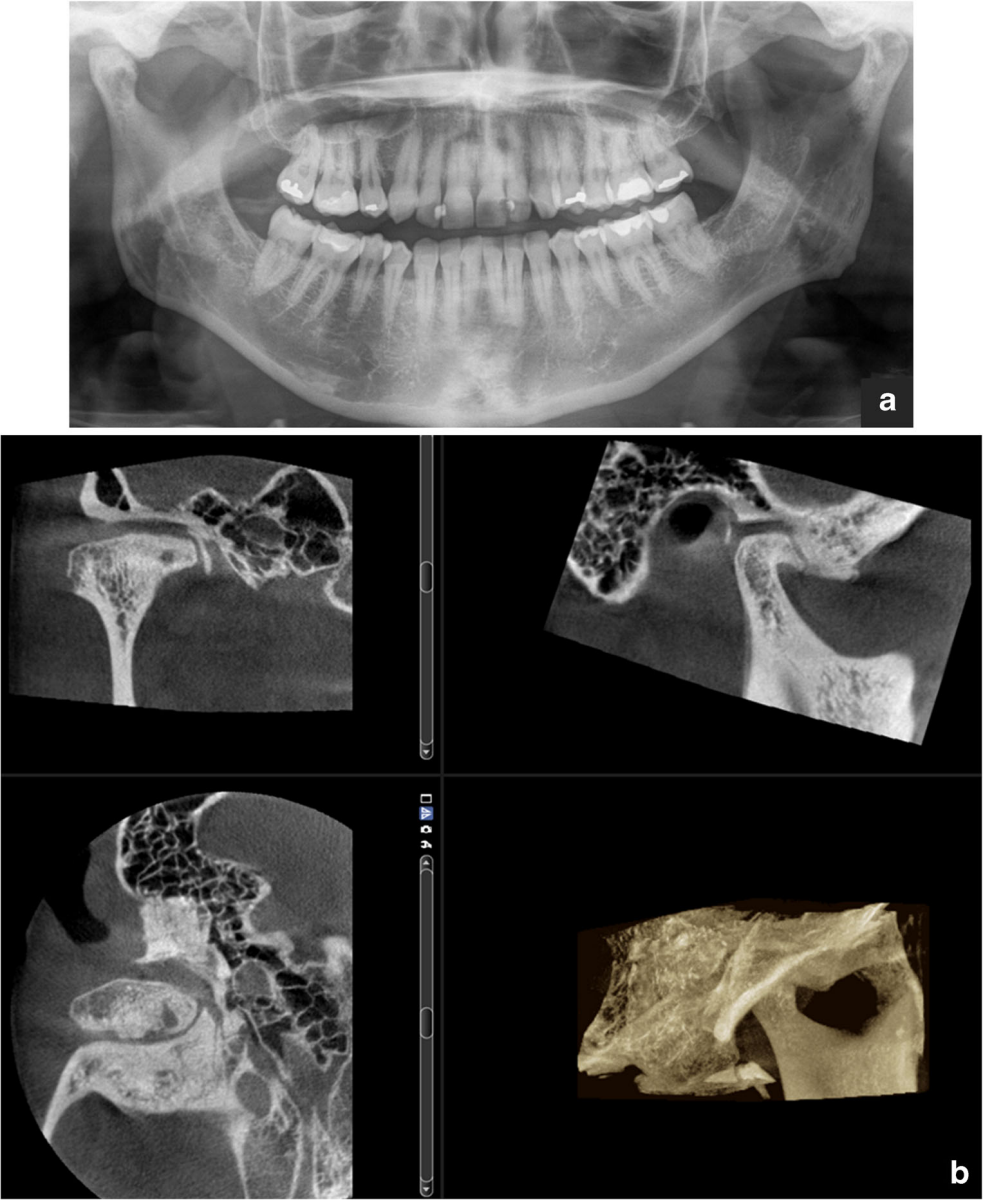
a Osteoarthrotic: flattening, osteophyte and subcortical cyst of the condyle, sclerosis in both the condyle and fossa, joint space narrowing: findings in a PTG visualised more clearly in b the CBCT (*right TMJ*)In the midfacial region the outlines of the maxilla and aeration of the sinuses should be evaluated to exclude pathology in these areas (Fig. 2). However, PTG has limited value for evaluation of sinus or midfacial pathology as only portions of these structures that are within the IL will be demonstrated [20]. The whole maxillomandibular bone must be evaluated for any signs of pathological conditions [9].
